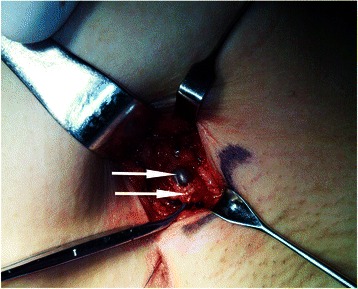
Identification of suspicious lymph node and SLN during surgery. The green arrow shows the methylene blue-dyed SLN, while the yellow arrow indicates the adjacent suspicious lymph node that was identified by preoperative ultrasound and wire localization. In this patient, final pathology confirmed that the SLN had no metastasis, while a metastasis was present in the suspicious lymph node.
